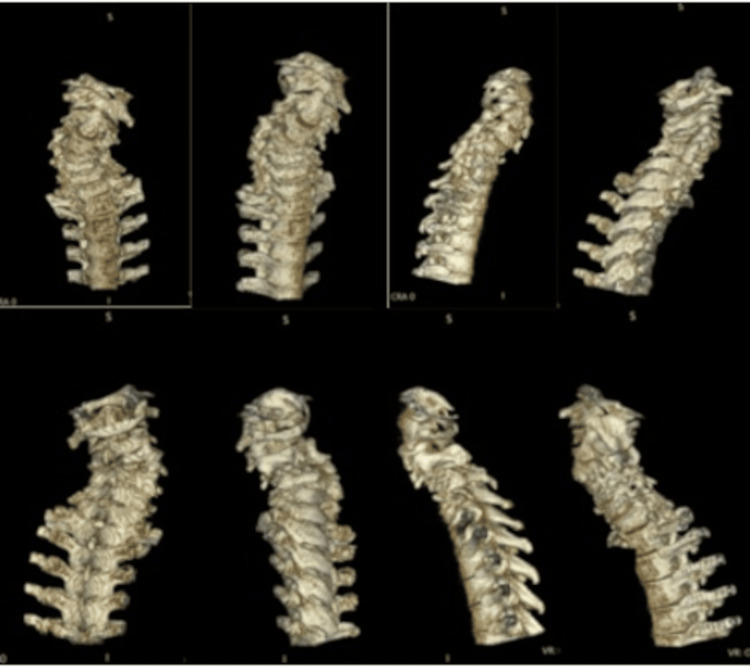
Three-Dimensional Reconstructed Cervical CT A 3D-reconstructed cervical CT was performed to visualize better the extent of the rotational component of the deformity.
